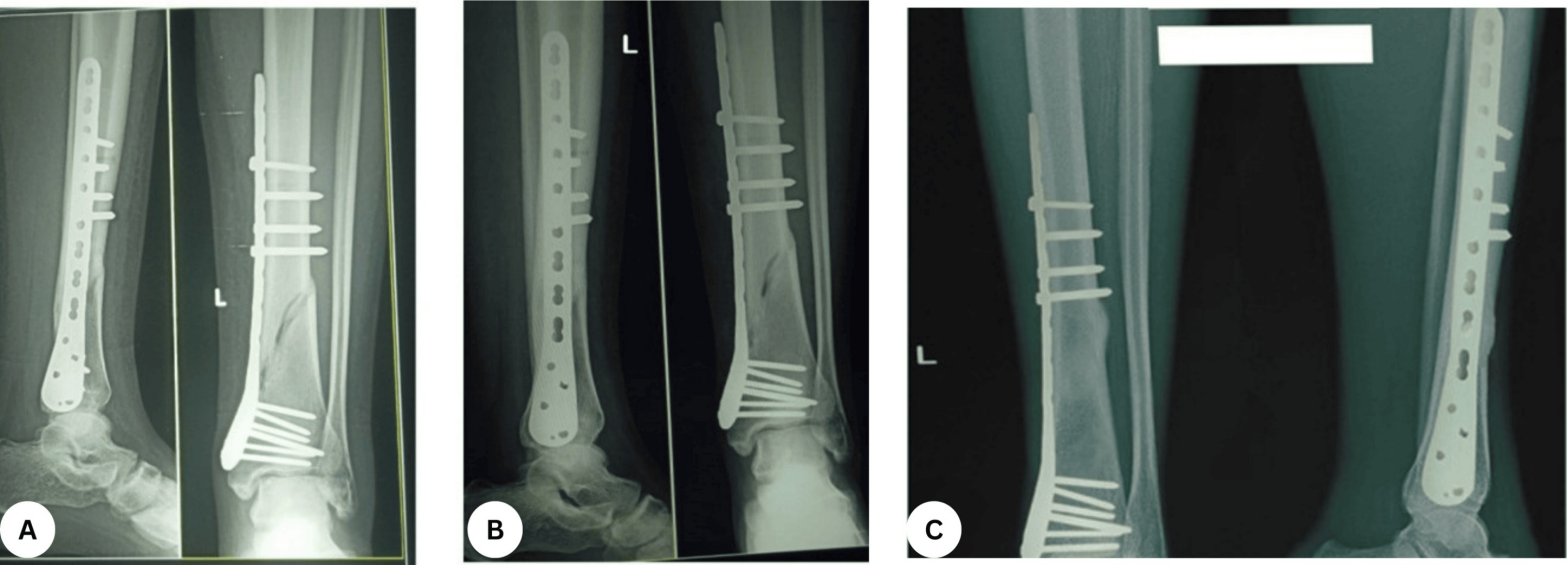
Serial postoperative radiographs after MIPO plating of a distal tibial fracture (AP and lateral views in each panel) (A) Early postoperative: plate spanning the metaphyseal–diaphyseal junction with clustered distal locking screws; alignment and length restored. (B) Intermediate follow-up: progressive callus with cortical bridging; hardware intact. (C) Late follow-up: circumferential cortical bridging consistent with solid union; no implant failure or loss of reduction. MIPO: minimally invasive plate osteosynthesis.
